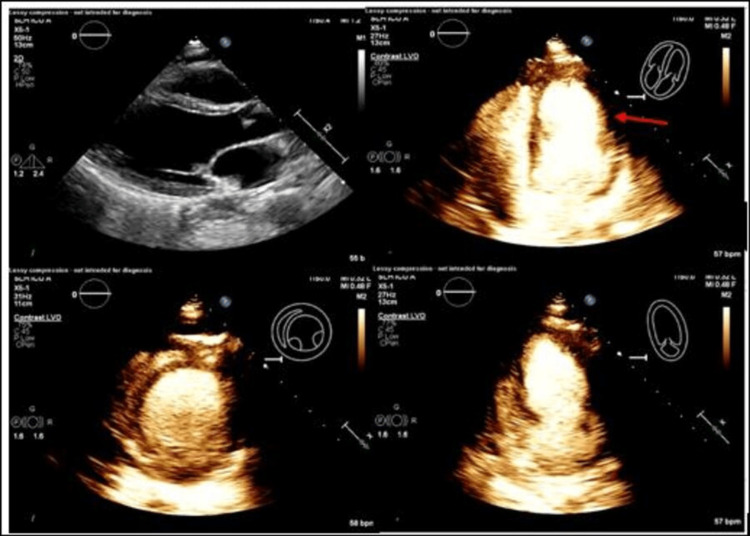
Echocardiogram with reduced ejection fraction concerning for stress cardiomyopathy. Echocardiogram revealed severely depressed left ventricular systolic function, with a calculated ejection fraction of 28% with apical and mid-anterior akinesis (red arrow) and preserved basal wall motion, suspicious of stress cardiomyopathy.
